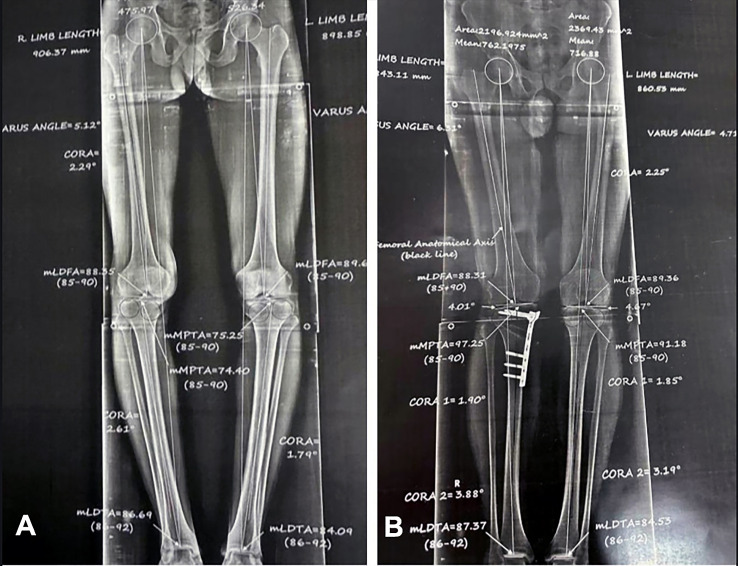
A standing whole lower limb radiograph (A) preoperative and (B) 3 months postoperative.
